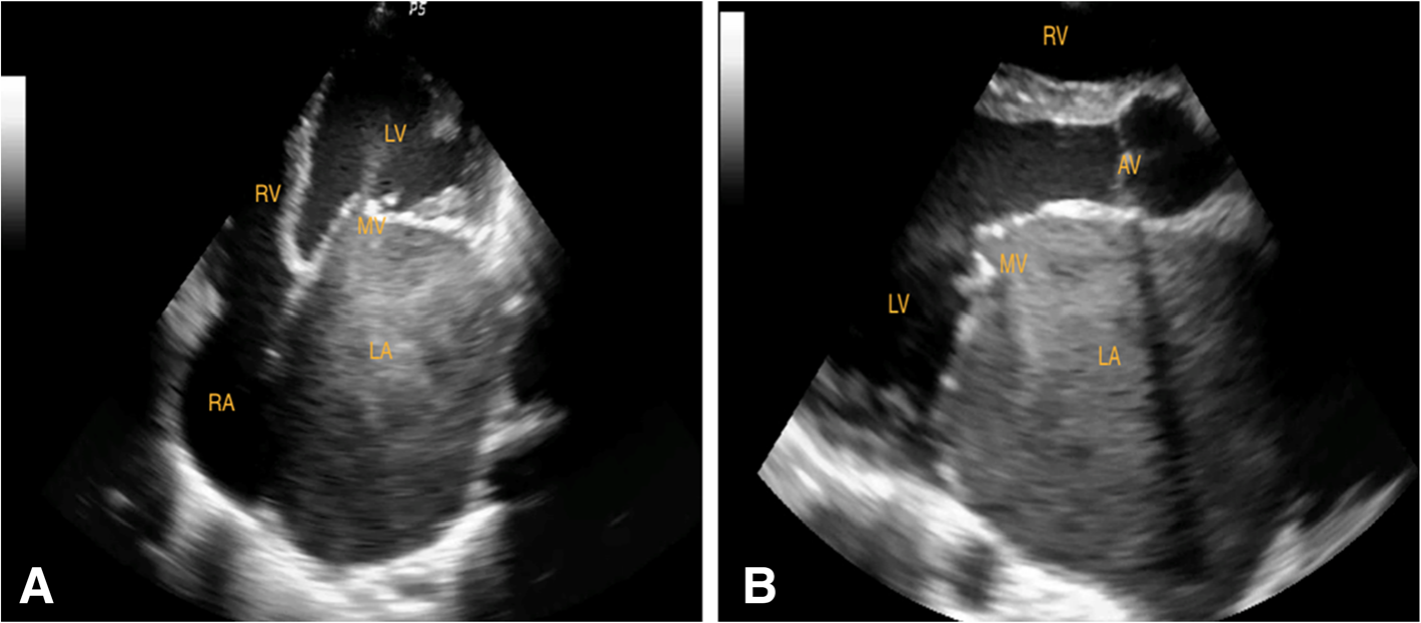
Echocardiographic frames from a 10-year-old-girl with rheumatic heart disease and severe mitral stenosis (MVA 0.51 cm^
